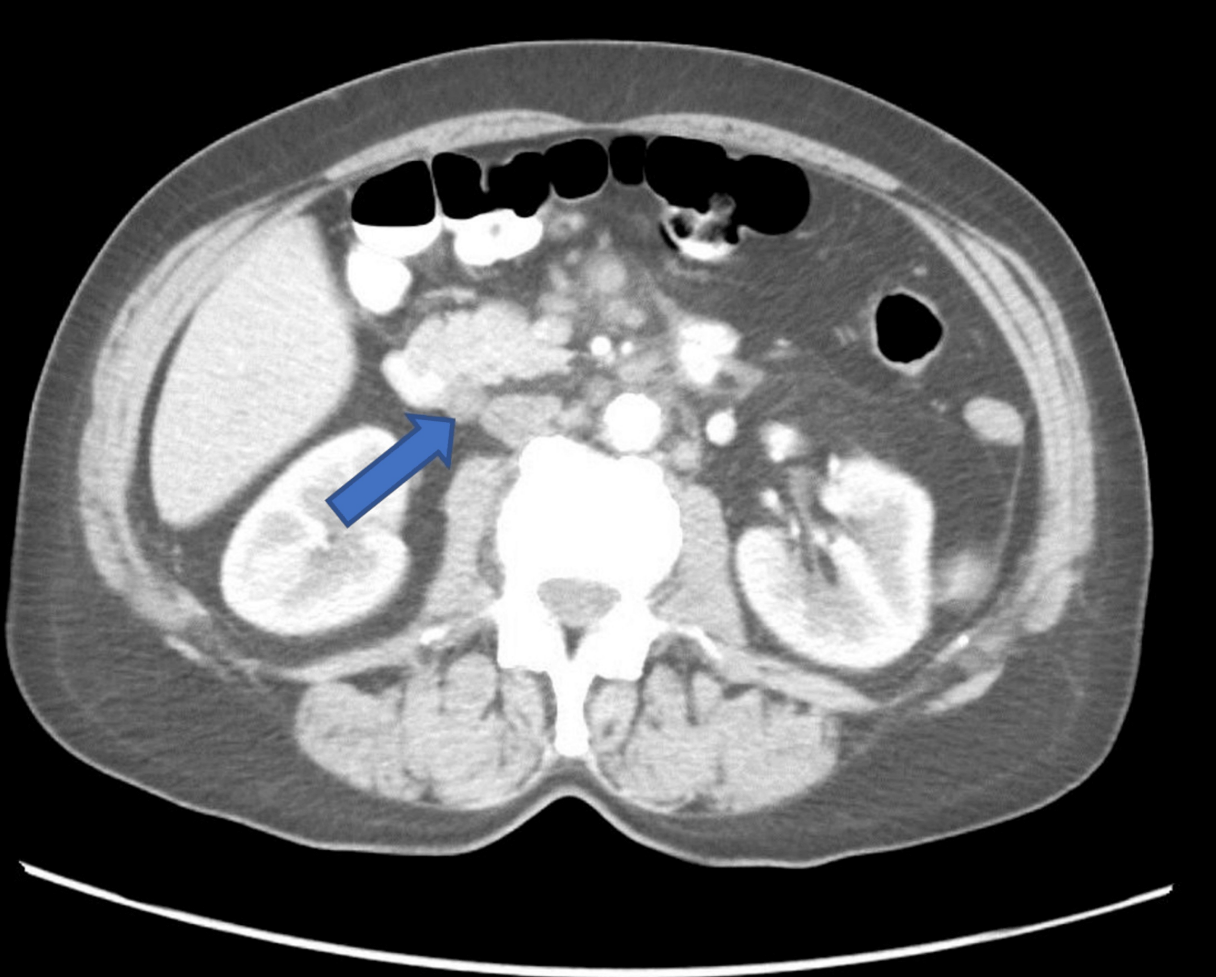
Abdominal axial CT showing enlarged paraaortic lymph nodes (arrow)
